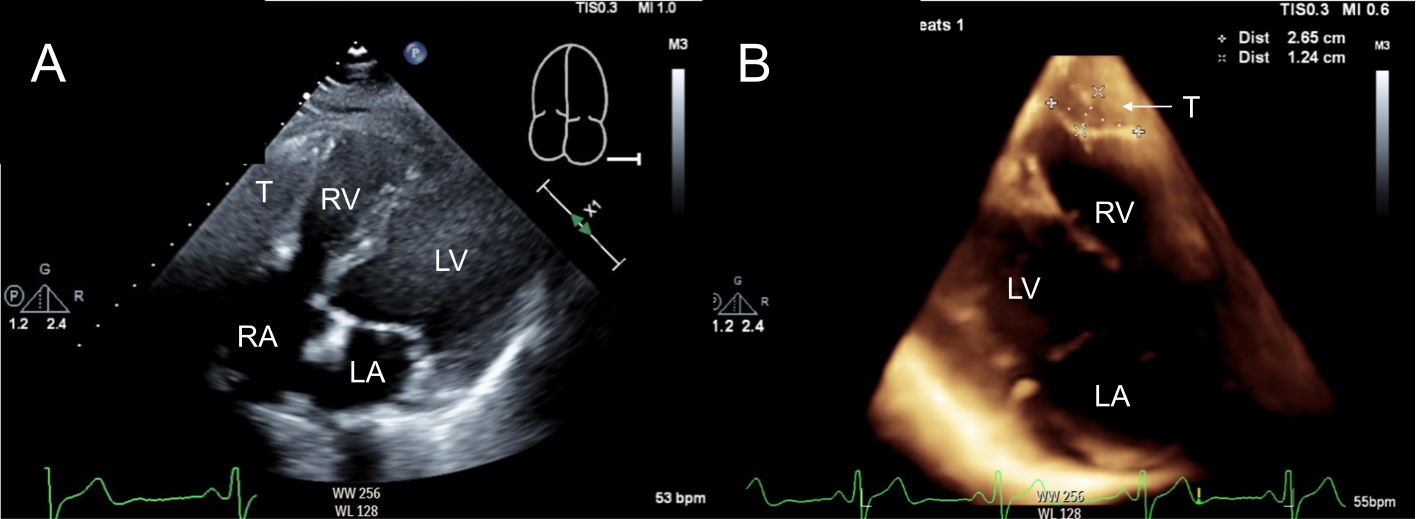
Preoperative transthoracic echocardiogram. a Two-dimensional four chamber view showing a non-mobile mass-like echo bright density protruding into the right ventricular free wall. The size and contiguous extension of the mass are unclear. No obvious pericardial effusion detected. b Three-dimensional view showing a well-demarcated mass in right ventricular free wall. T: Tumor, LV: Left Ventricular Cavity, RV: Right Ventricular Cavity, LA: Left Atrium, RA: Right Atrium
